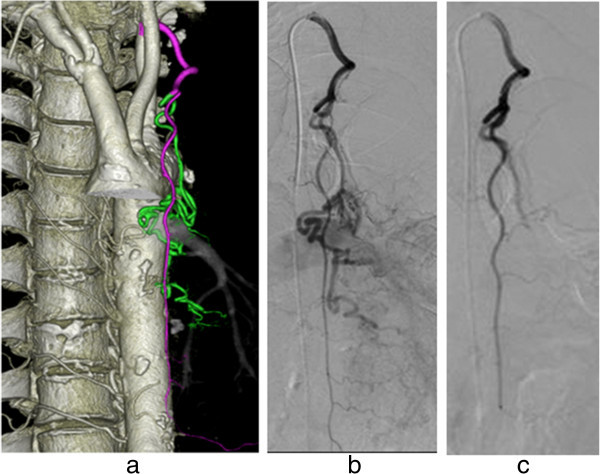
A case of hemoptysis. Hemorrhage-responsible arteriogram created by CT during thoracic aortography depicts the left bronchial artery (green) branching from the left intra-thoracic artery (purple) (a). A microcatheter was advanced via a preshaped catheter and inserted into the left intra-thoracic artery, followed by selective embolization of the left bronchial artery with gelatin sponge particles as shown in the angiography before (b) and after (c) embolization.
